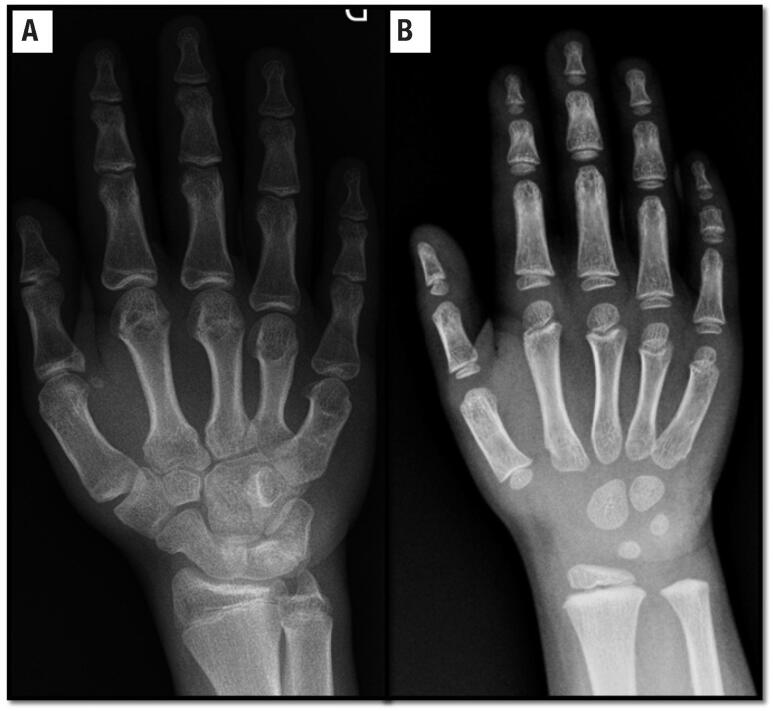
Hand radiographs of children carrying *NPR2* and *IHH* mutations. (A) Hand radiograph of a patient with heterozygous mutation in *NPR2.* Shortening of metacarpal is clearly observed. (B) Hand radiograph of a child with heterozygous mutation in *IHH* showing the shortening of the middle phalanx of the 5^th^ finger with cone-shaped epiphyses.
